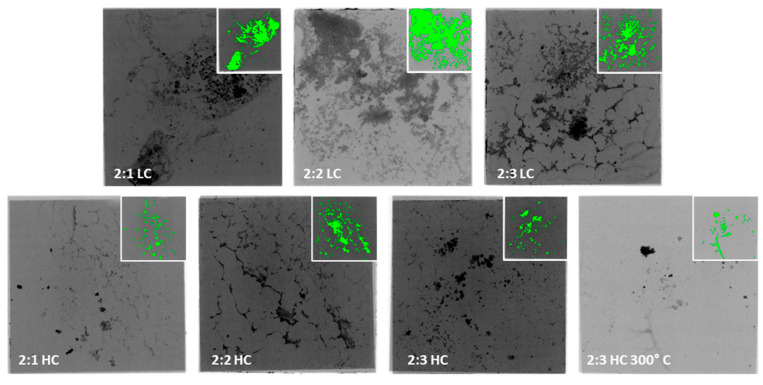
X-ray images of the TIM material series tested, showing an example of image thresholding.
